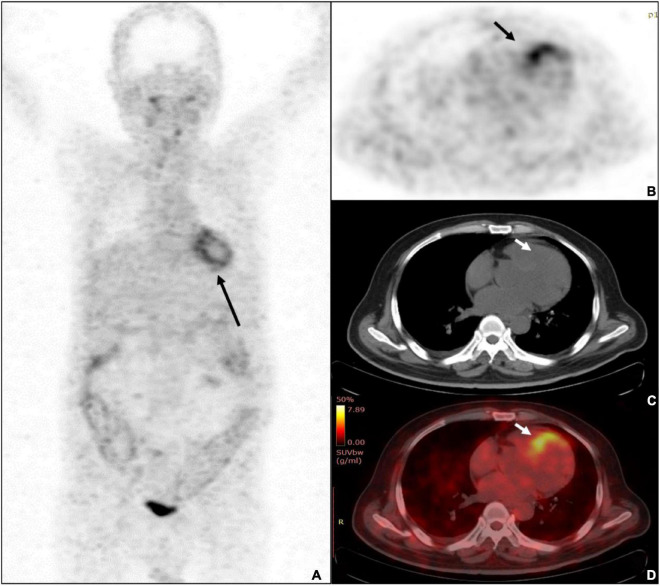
A 68-years-old male patient was diagnosed with ulcerative colitis and diabetes mellitus. (A) The whole-body MIP of Al^18^F-NOTA-FAPI-04 PET imaging demonstrated diffused uptake pattern in LV (black arrow) and diffused uptake with colons. (B) The PET imaging showed the highest uptake of SUVmax was 7.0 (black arrow). (C) Unfused CT image of the heart. (D) The fusion imaging showed the septal wall of prominent uptake of FAPI-04.
